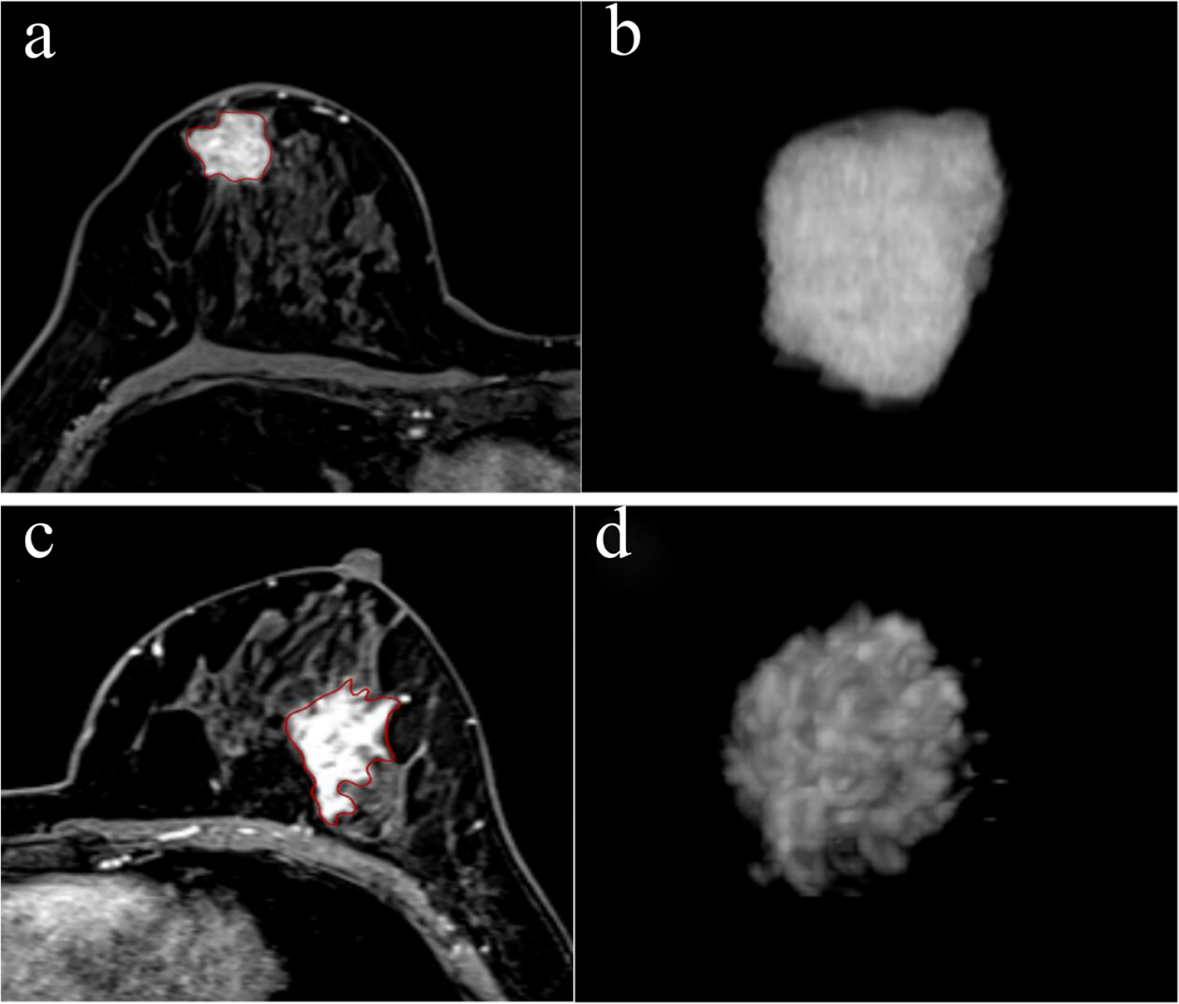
Segmentation of breast lesions on CE-MRI. Images a-b show the right invasive breast cancer that was non-pCR after NAC. Images c-d show the left invasive breast cancer that was pCR after NAC. a, c Segmentation of breast lesions on CE-MRI. b, d 3D imaging of VOIs
